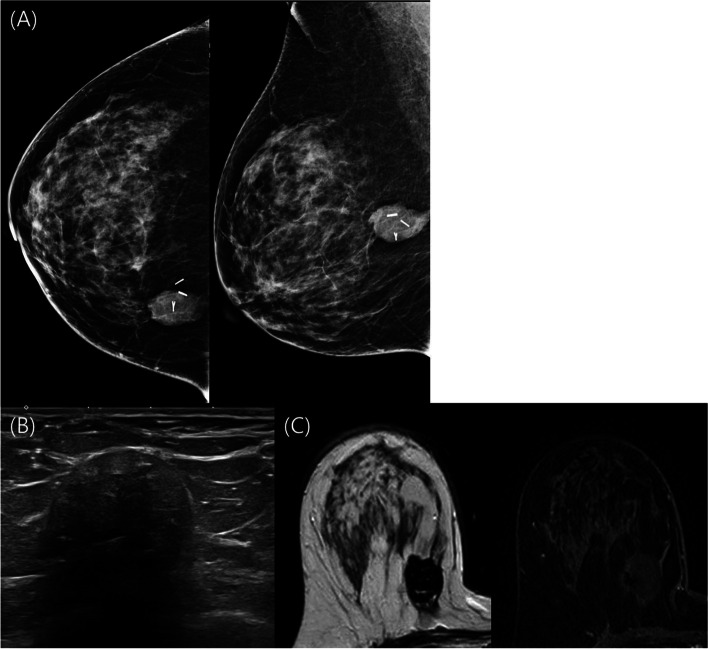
Follow-up imaging after 24 months of a 49-year-old woman who underwent oncoplastic BCS with ADM. The ADM was observed as A a circumscribed high-density mass on mammography and B a heterogeneous echogenic mass with posterior acoustic shadowing. The evaluation of the deep posterior margin of the excision cavity was limited by shadowing. The ADM showed low signal intensity on T2-weighted imaging and no enhancement on contrast-enhanced T1-weighted imaging (C)
